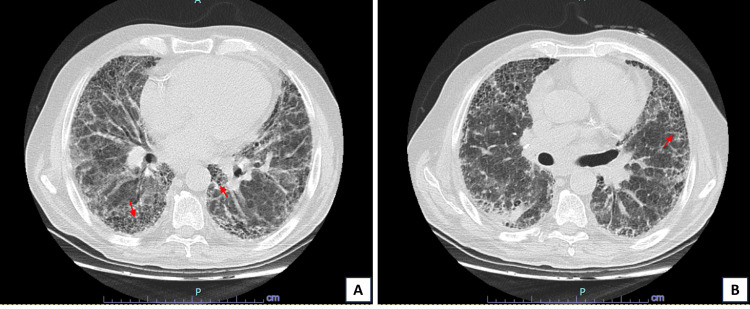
Axial images of chest CT two years later during hospitalization for an infectious complication. (A) and (B) with evident worsening of previously identified fibrotic changes (red arrows) indicative of advanced-stage pulmonary fibrosis.
